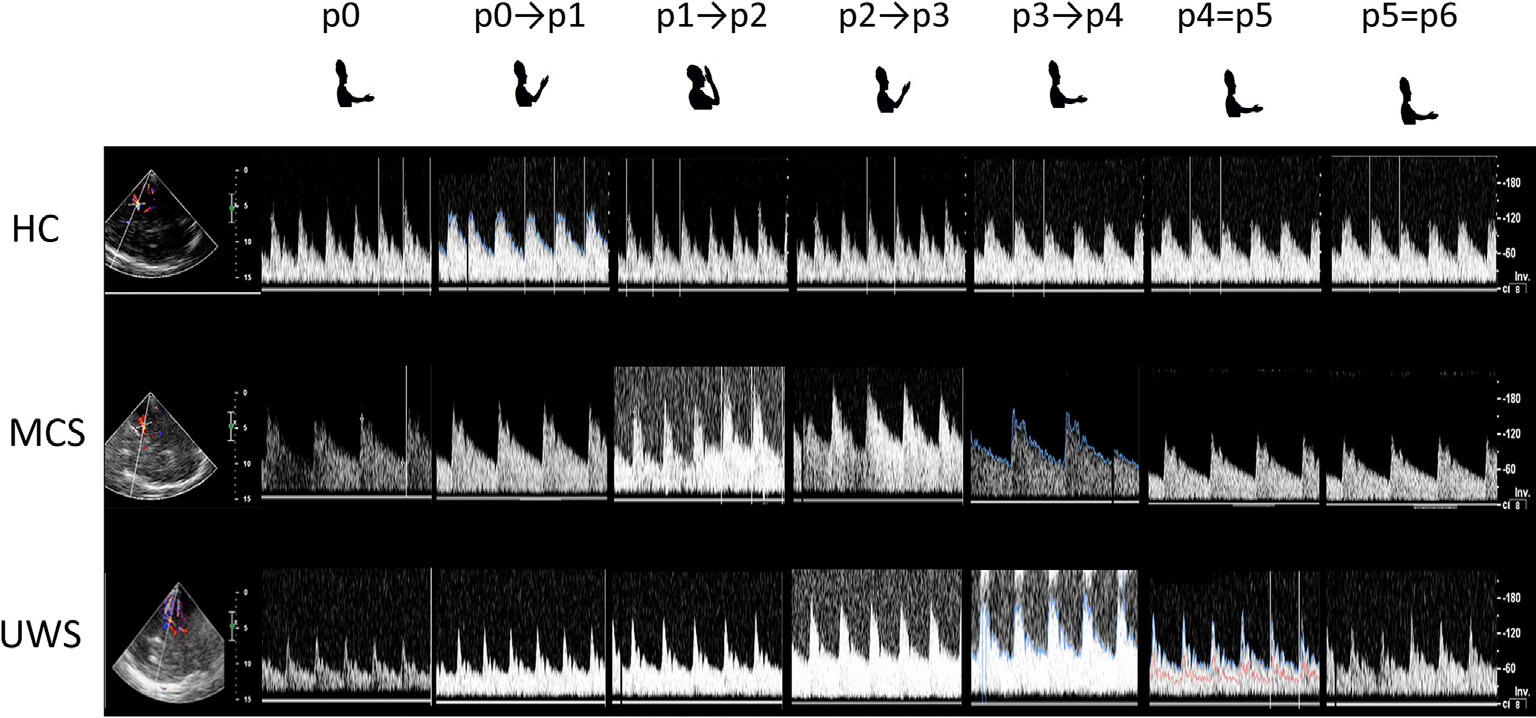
Shows the transcranial Doppler waveforms (on the right) with color Doppler (on the left) from left middle cerebral artery during passive mobilization across the six hand positions (p) explored in a representative subject for each group [healthy controls, minimally conscious state (MCS), and unresponsive wakefulness syndrome (UWS)]. Both insonation depth (left vertical bar) and flow velocity (centimeter per second, right vertical bar) are provided.
